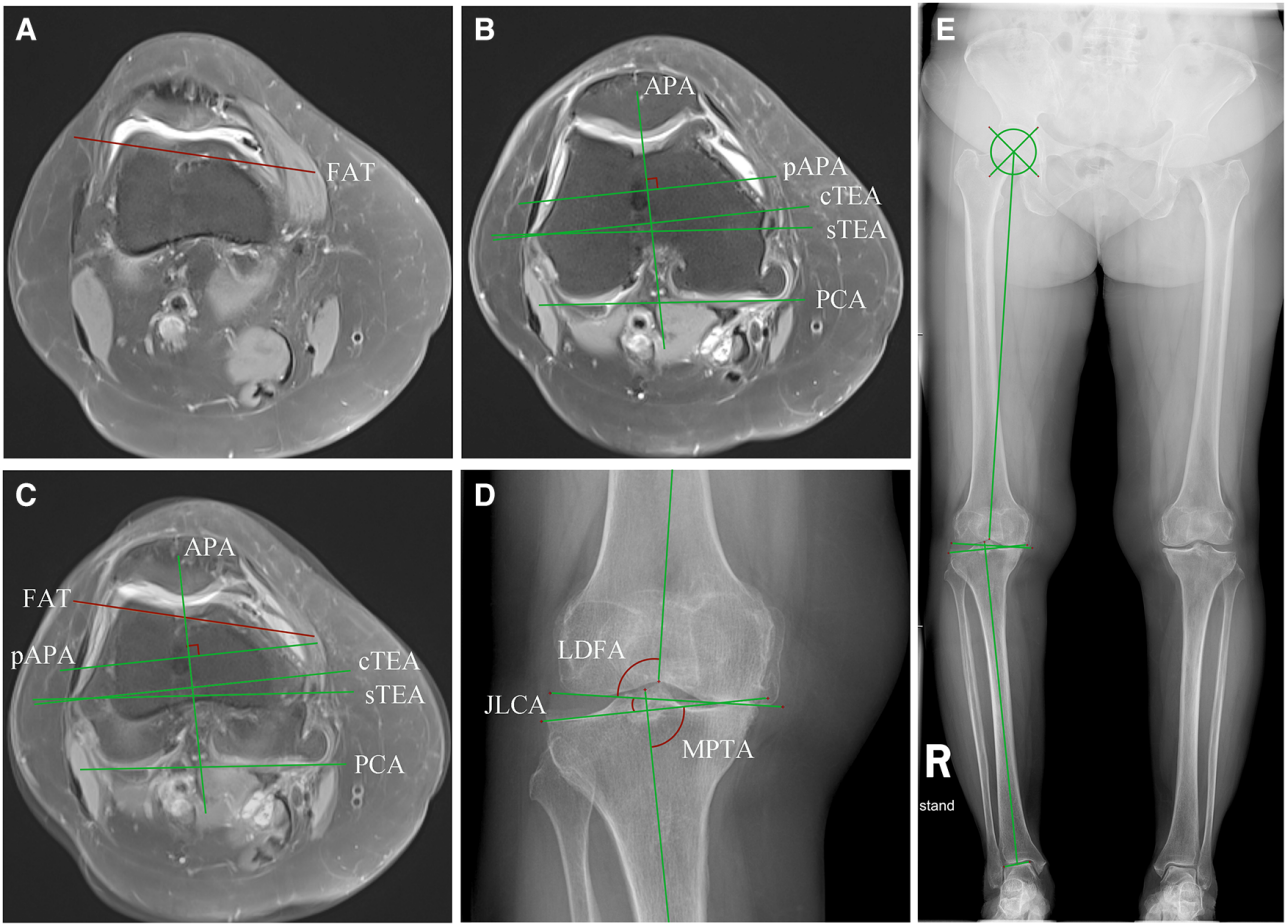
Measurement of the distal femoral rotational axes on MRI and lower limb alignment angles on radiography. (A) The FAT line was drawn on the transverse plane of MRI. The slice was usually two slices apart from the upper edge of the trochlear cartilage; (B) The cTEA, the sTEA, the PCA, the APA, and the line pAPA were identified on another slice of the transverse MRI. Note that the cartilage wear of the posterior femoral condyle and the trochlear could sometimes affect the position of the PCA and pAPA. (C) (A) and (B) were merged and the angles between the indirect rotational reference axes and the transepicondylar axes were measured. (D) The JLCA, the LDFA, the MPTA, and the mHKA were measured on a knee radiograph derived from a double-limb stance radiograph (E). The position of the lower limb alignment axes and angles are shown in (E).
